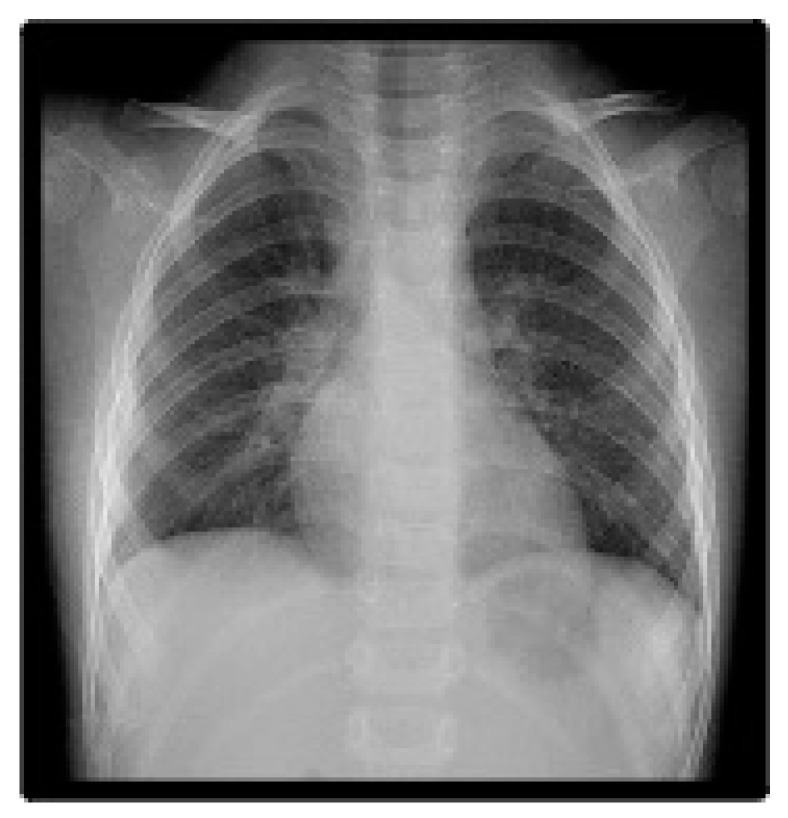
Follow-up frontal chest X-ray (20 days after hospital discharge) demonstrates full recovery.
